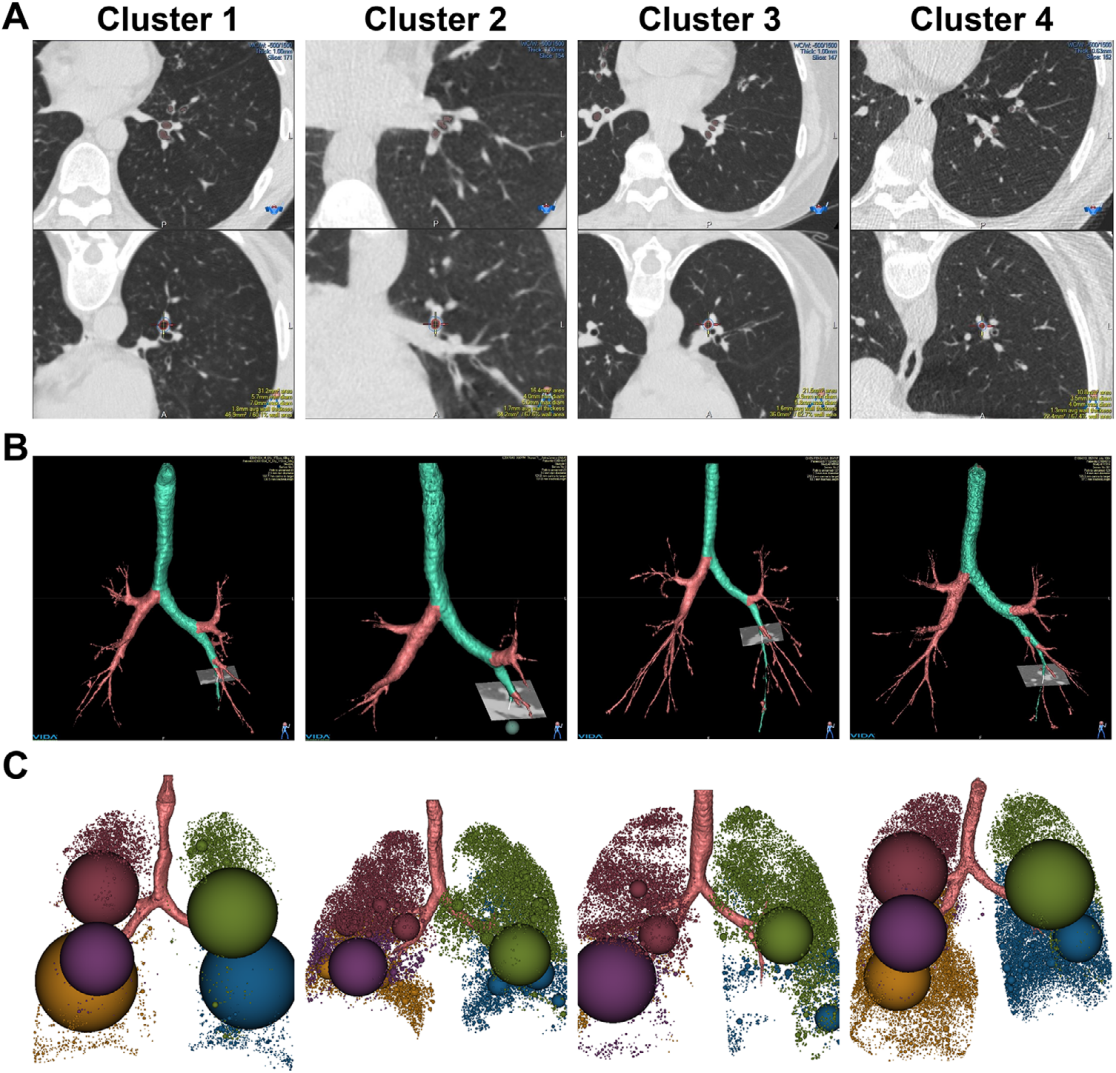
HRCT imaging and analysis of airway and emphysematous features across four clusters. (A) Upper row displays cross‐sectional HRCT images of the third‐order bronchus (LB10) for luminal area assessment; lower row shows airway wall thickness measurements. (B) Tracheobronchial tree reconstructed using VIDA Workstation (version 1.2). (C) Density mask method quantifies air trapping and emphysematous lesions, with color‐coded spheres indicating low attenuation areas (<−856 HU) by pulmonary lobe.
